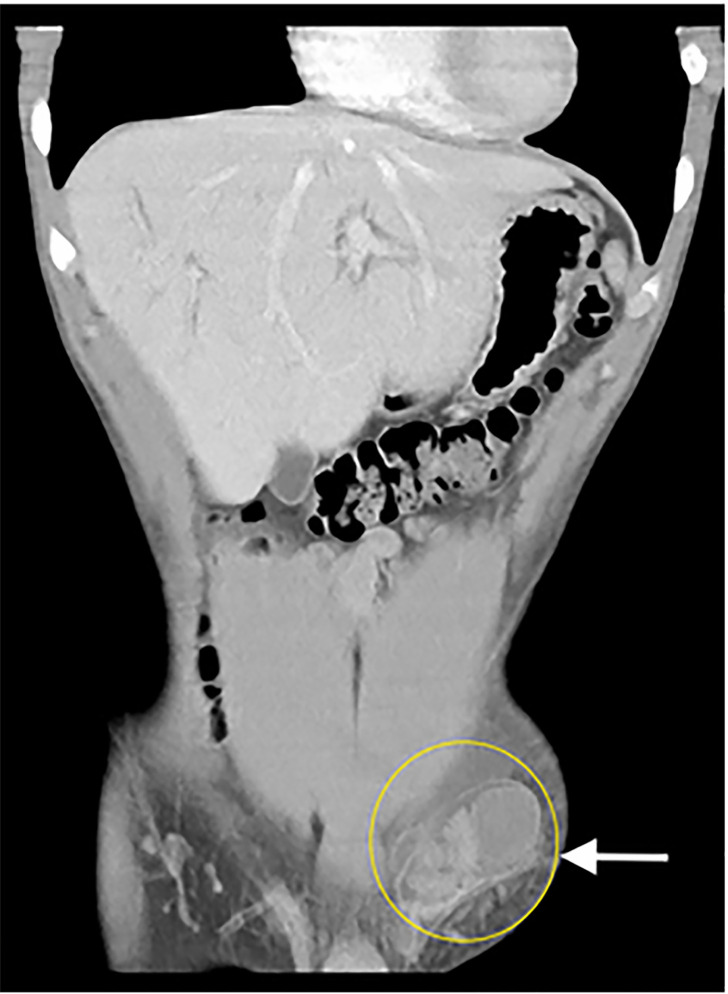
Coronal reconstruction computed tomography demonstrating the cryptorchid
